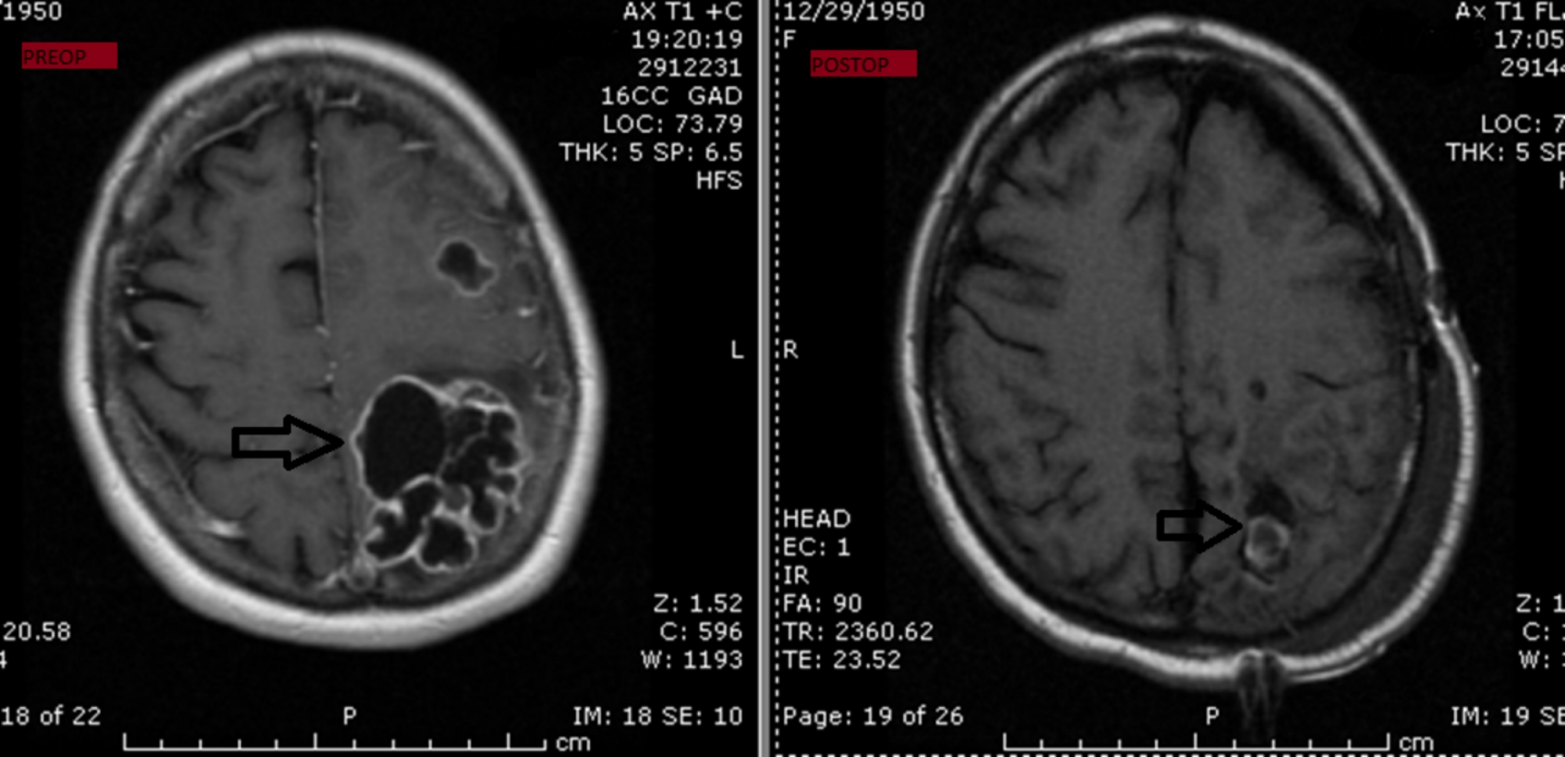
Preoperative and postoperative magnetic resonance imaging of the brain revealing a ring-enhancing mass in the left parieto-occipital lobe.
